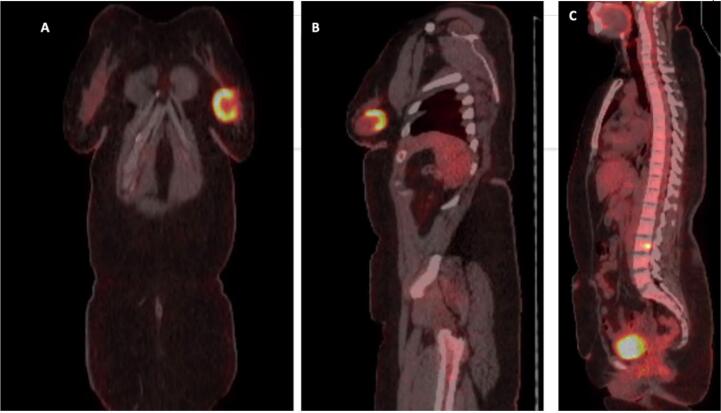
PET-CT scan coronal (A), and sagittal (B, C) showing interval increased size and uptake of the previously identified left breast mass lesion, measuring 4.8 cm compared to 2.2 cm with SUV max 10 compared to 8 previously, consistent with disease progression. And (C) showing newly apparent focal intense metabolically active bony lesion in the pedicle of L-3 lumbar vertebra with SUV max 6.5, probably bone metastasis.
